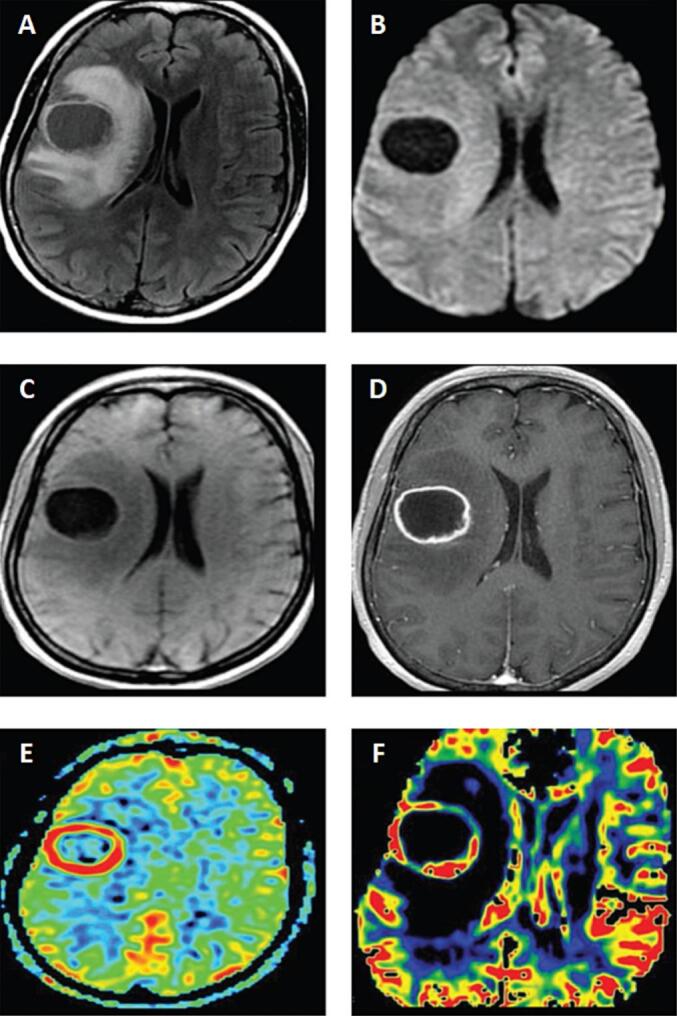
[A] Axial FLAIR and [B] axial DWI demonstrate a hypointense lesion with significant perilesional vasogenic edema and no restricted diffusion. [C] Axial precontrast T1WI and [D] axial postcontrast T1WI demonstrate a large irregular and thick ring-enhancing lesion in the right frontal lobe. [E] Perfusion without contrast, ASL, shows increased rCBF in the periphery of the lesion. [F] DSC perfusion MRI shows significantly elevated CBV at the periphery of the lesion.
